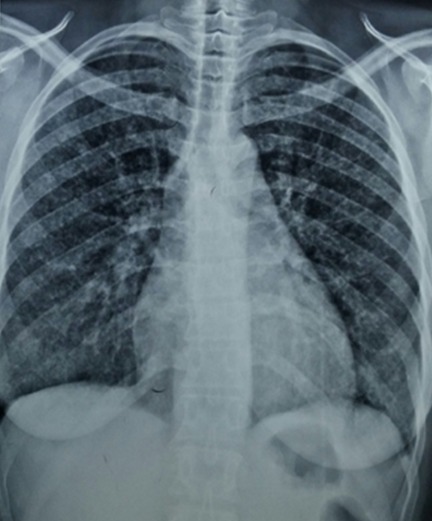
Radiographie du thorax de face montrant des micronodules disséminés de façon diffuse sur les deux champs pulmonaires associés à des opacités réticulaires
